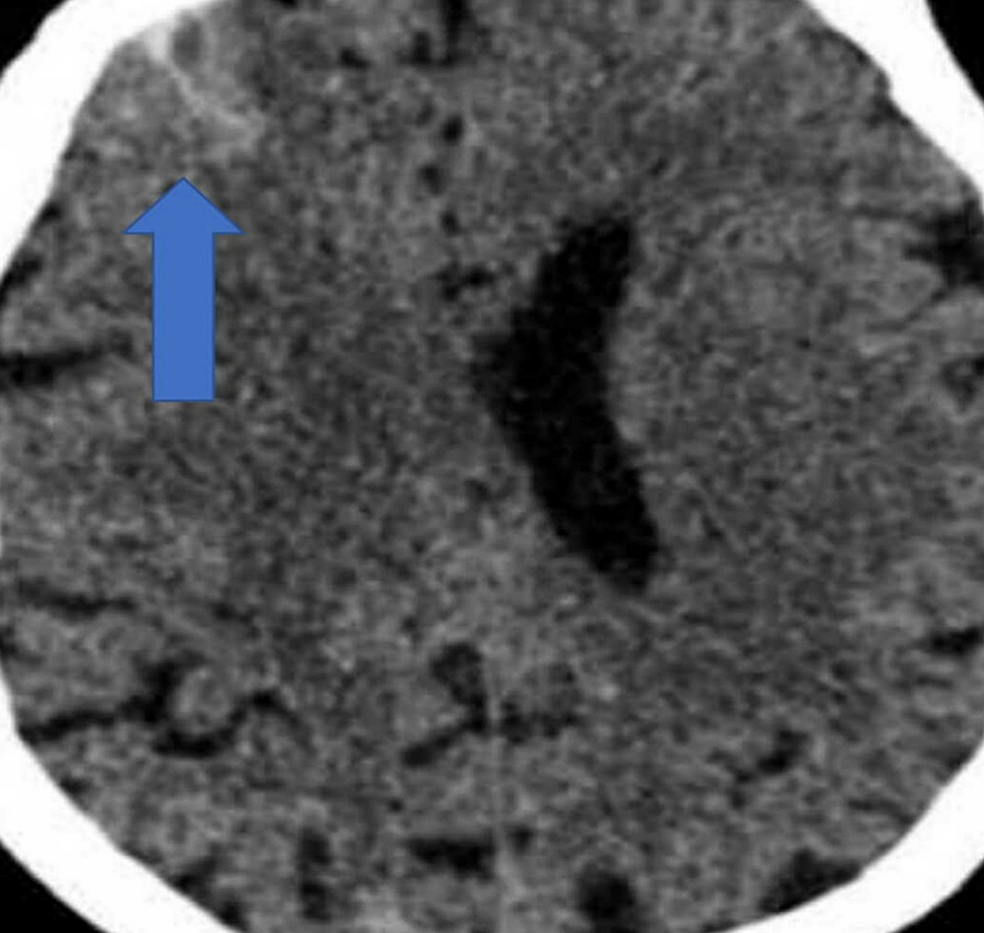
Non-contrast CT head axial view showing subarachnoid haemorrhage (arrow) in the right frontal, superior parietal regions
